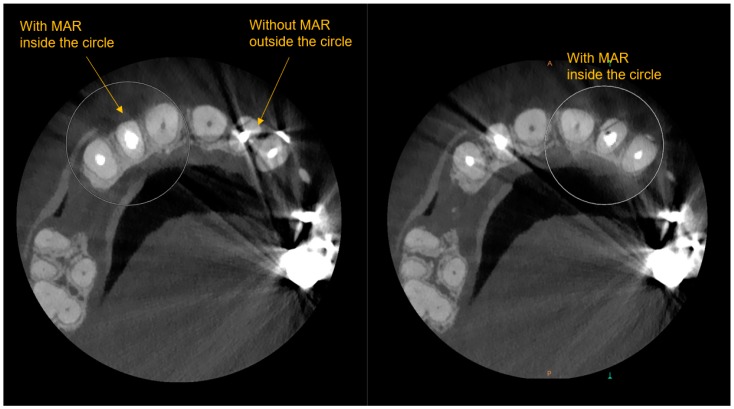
Axial views of CBCT with and without Metal artifact reduction (MAR) - (Carestream CS9600 MAR).
